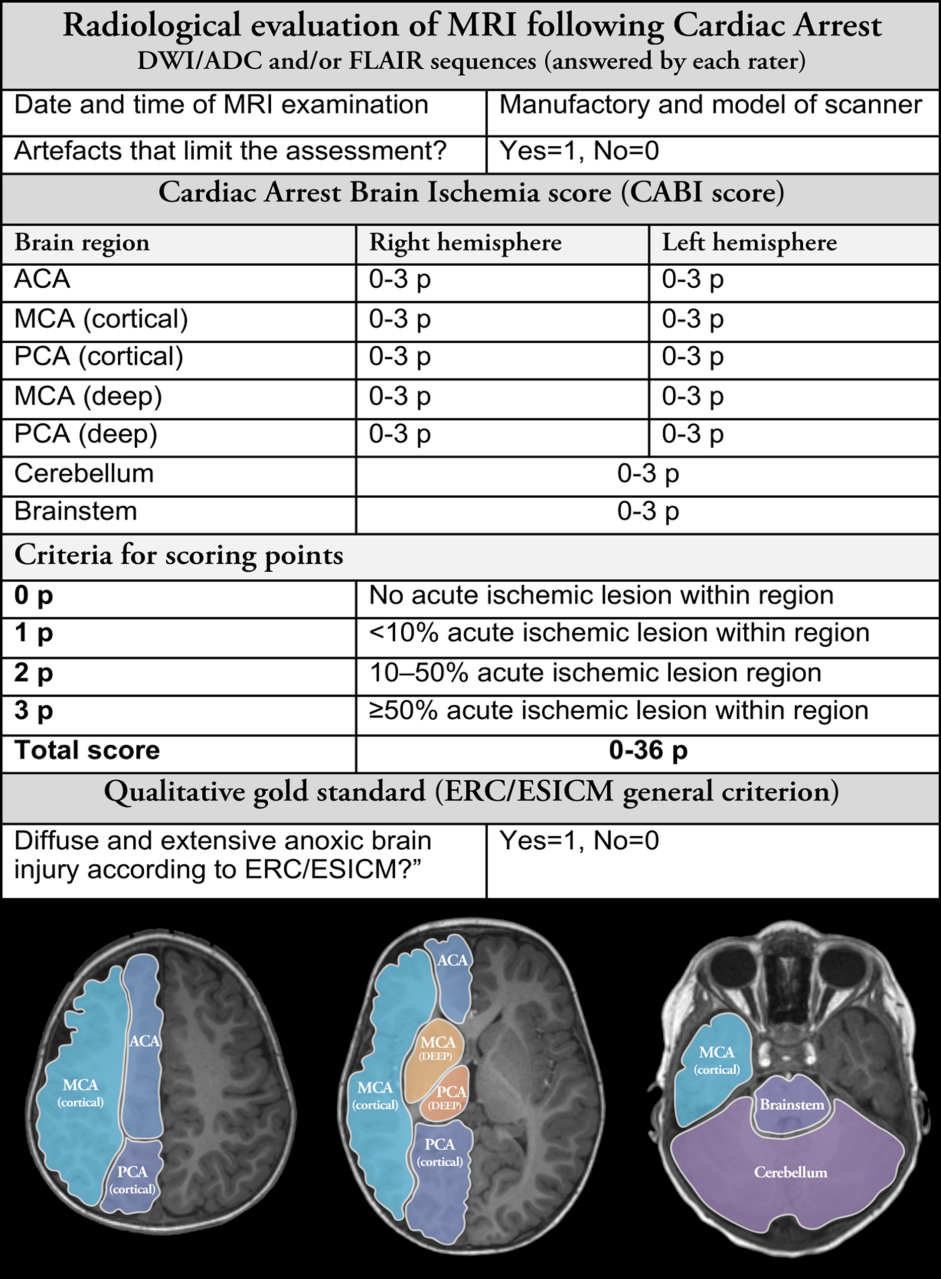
The radiological checklist for brain MRI evaluation. The figure shows the checklist for brain MRI evaluation used by the four raters. The checklist includes the semi-quantitative Cardiac Arrest Brain Ischemia (CABI) score and the qualitative assessment as per ERC/ESICM recommendations. The CABI score is based on vascular/anatomical regions and has maximum of 36 points: anterior cerebral artery, middle cerebral artery (cortical respectively deep), posterior cerebral artery (cortical respectively deep), cerebellum and the brainstem.*DWI; Diffusion-Weighted Imaging*,* ERC; European Resuscitation Council*,* ESICM; European Society of Intensive Care Medicine*,* FLAIR; Fluid-Attenuated Inversion Recovery*,* MRI; Magnetic Resonance Imaging*
